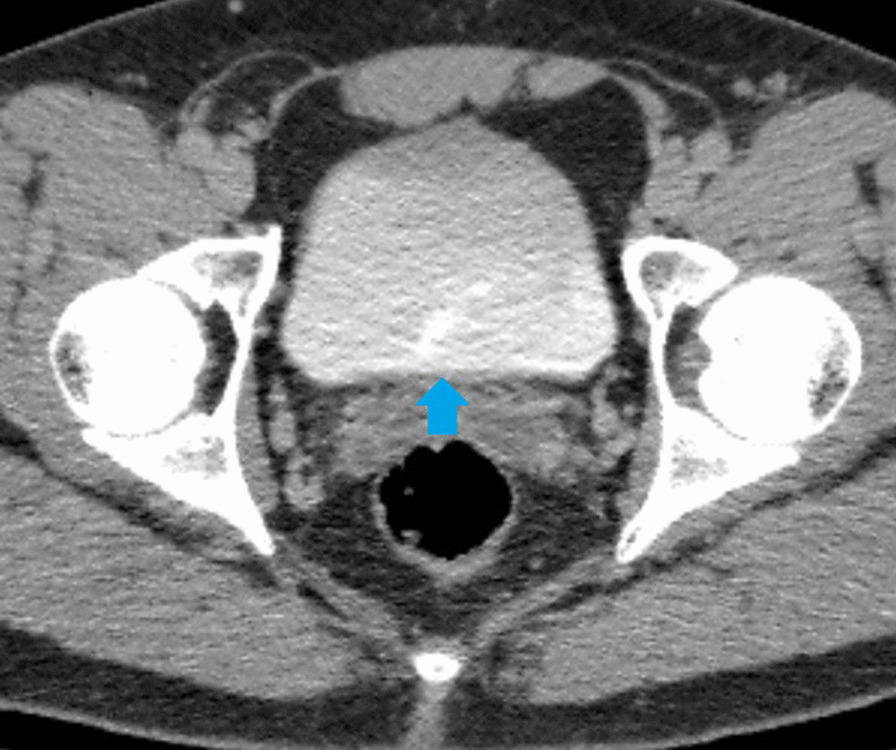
A contrast-enhanced computed tomography axial section delayed phase image at the level of the urinary bladder demonstrates normal opacification of the bladder (blue arrow)
